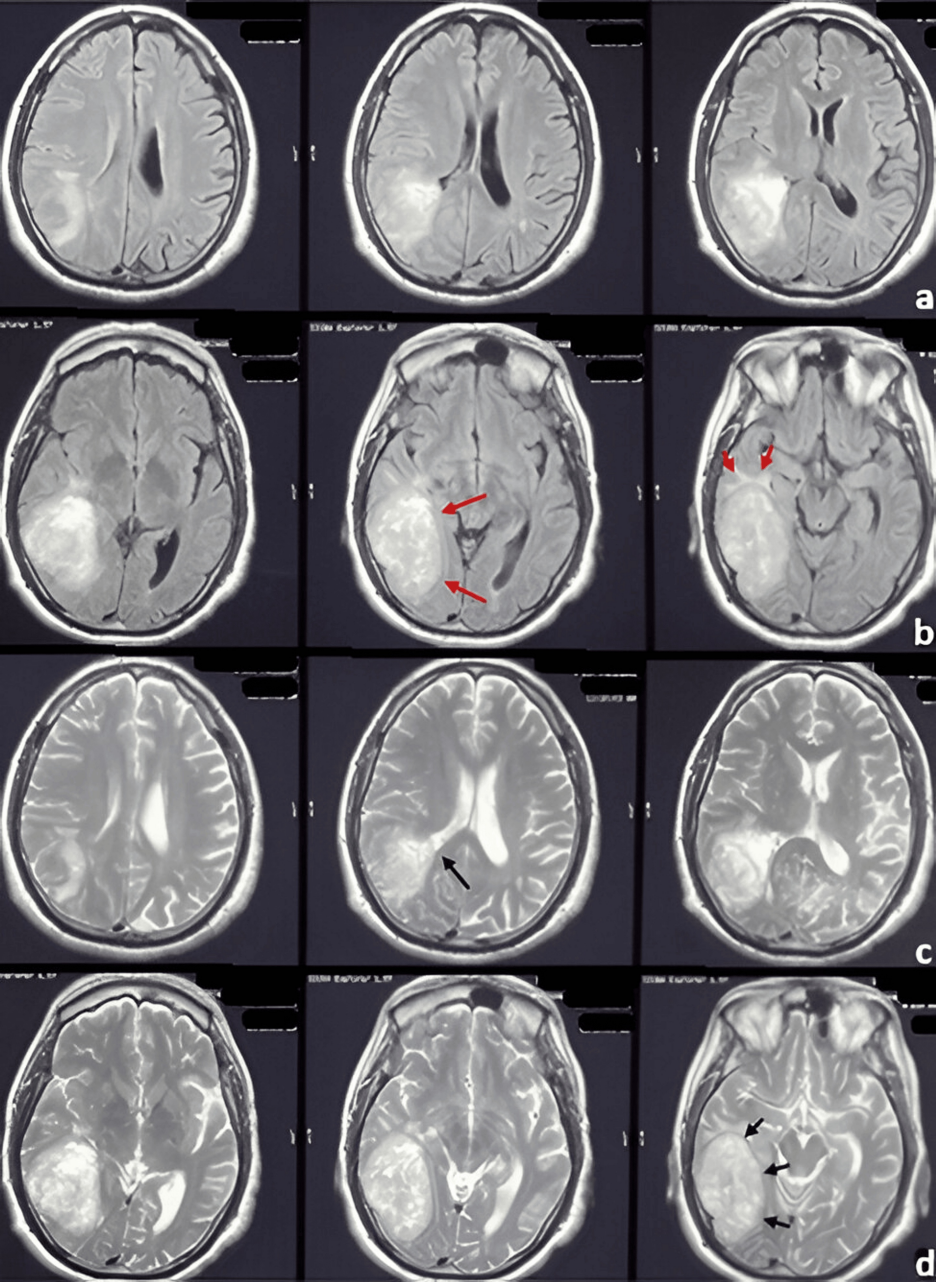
MRI demonstrating a right temporal gliosarcoma (a) Contrast-enhanced T1-weighted MRI showing three axial sections with a right temporal lesion demonstrating heterogeneous enhancement following contrast administration. (b) Contrast-enhanced T1-weighted MRI showing three axial sections with well-defined margins in the medial portion of the lesion (long red arrows) and the presence of predominantly temporal perilesional edema (short red arrows). (c) T2-weighted MRI showing three axial sections demonstrating a close relationship between the lesion and the occipital horn of the right lateral ventricle (long black arrow). (d) T2-weighted MRI showing three axial sections in which a well-defined solid-appearing lesion is observed (short black arrows). MRI: Magnetic resonance imaging
